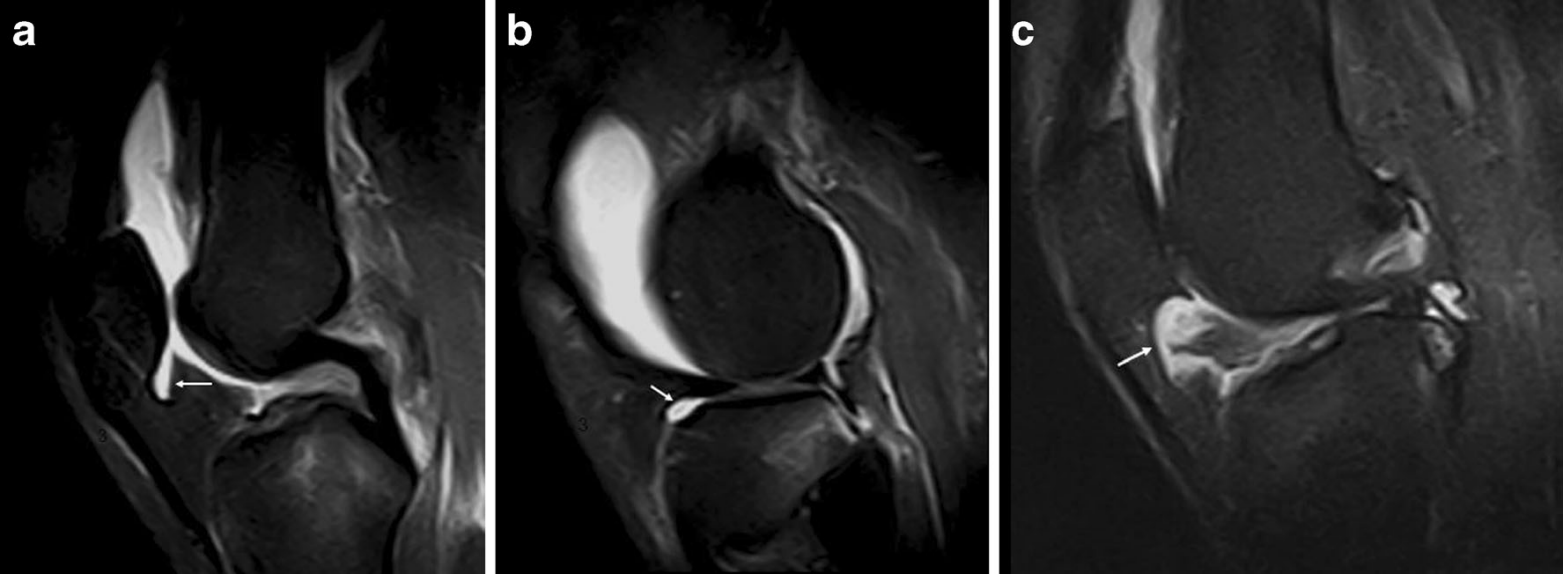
Suprahoffatic recess and infrahoffatic recess. Sagittal proton density with fat saturation MRI images show fluid in suprapatellar pouch, suprahoffatic recess (*arrow*, a) and infrahoffatic recess (*arrow*, b); a communication between the vertical and horizontal recesses may be present (*arrow*, c)
